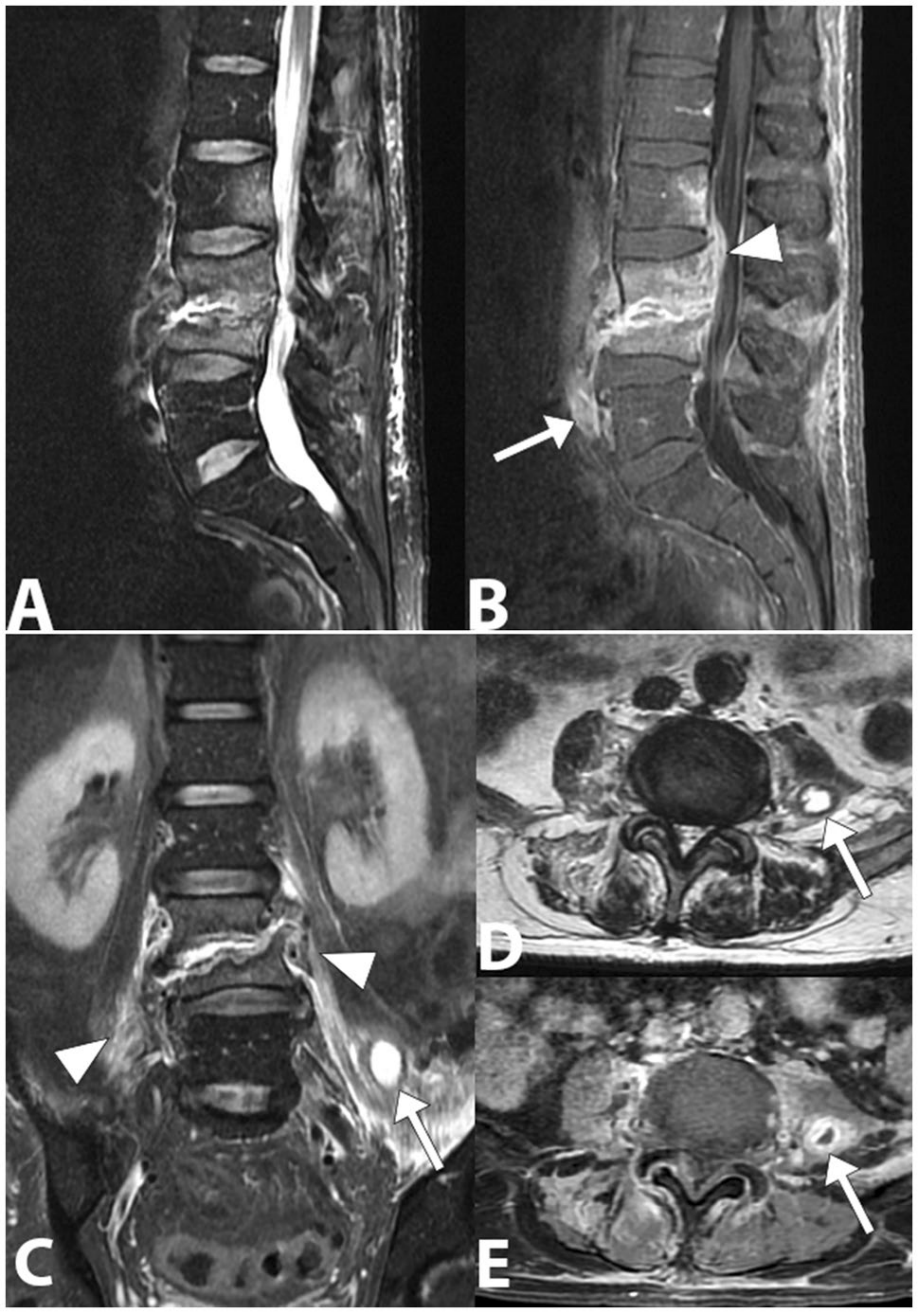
Gram-negative (Escherichia coli) spondylodiscitis. Sagittal lumbar spine MRI image (a) showing a  high bone marrow signal and partial collapse of the vertebral bodies L3 and L4, destruction of the L3–L4 disc and loss of the definition of the end plate on both sides of the disc. In the T1-weighted sagittal image obtained with saturation of the fat signal and after the injection of the contrast medium (b), a better definition of the involvement of the paravertebral (arrow) and epidural space with narrowing of the vertebral canal (arrowhead) can be observed. The posterior L2 vertebral body is also involved. In coronal STIR image (c), axial T2- weighted (d) and axial T1- weighted with fat saturation and gadolinium (e), edema and inflammatory exudate can be observed in the paravertebral soft tissue (arrowheads) and abscesses in the left psoas muscle (arrow)
